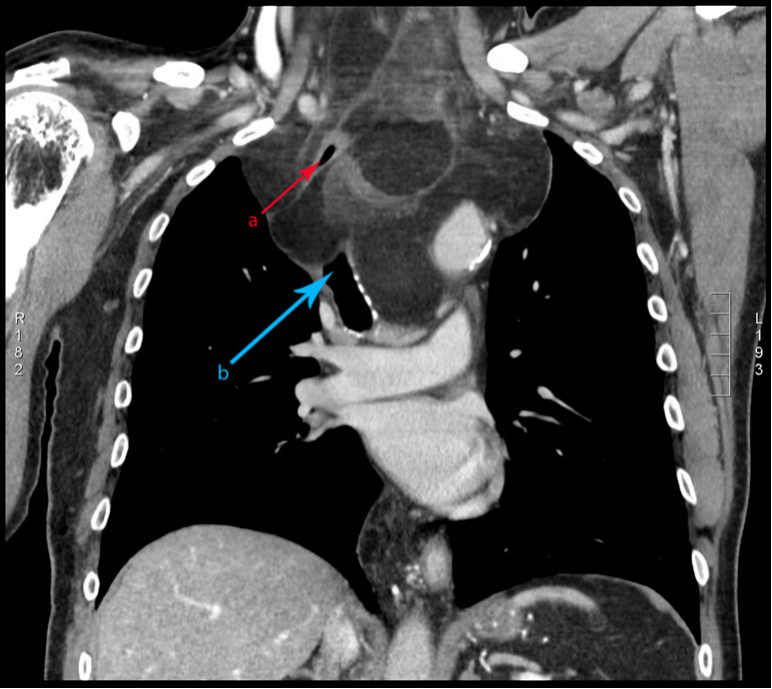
Coronal CT images of well-differentiated mediastinal liposarcoma. (a) indicates the position of the esophagus and (b) indicates the position of the trachea.
